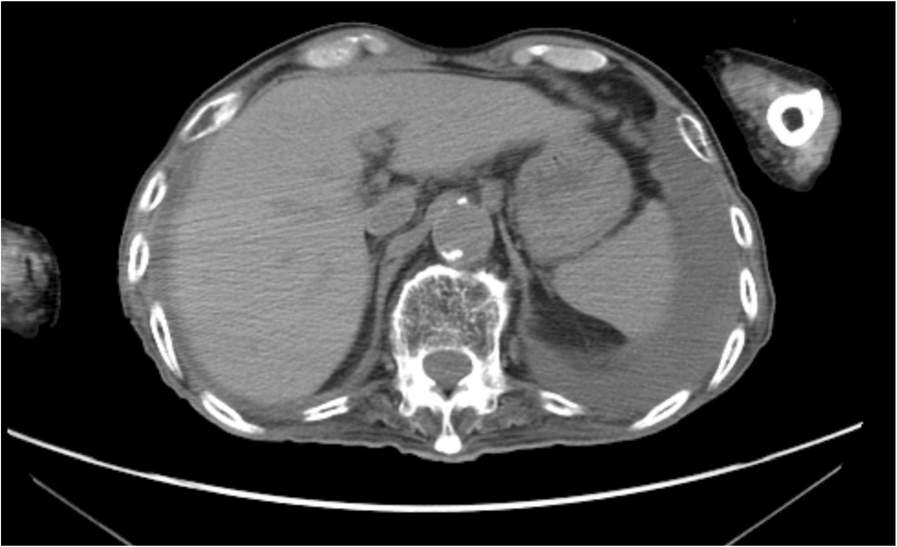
Follow-up computed tomography on hospital day 14 showed resolution of hepatic portal venous gas and air within the stomach wall
